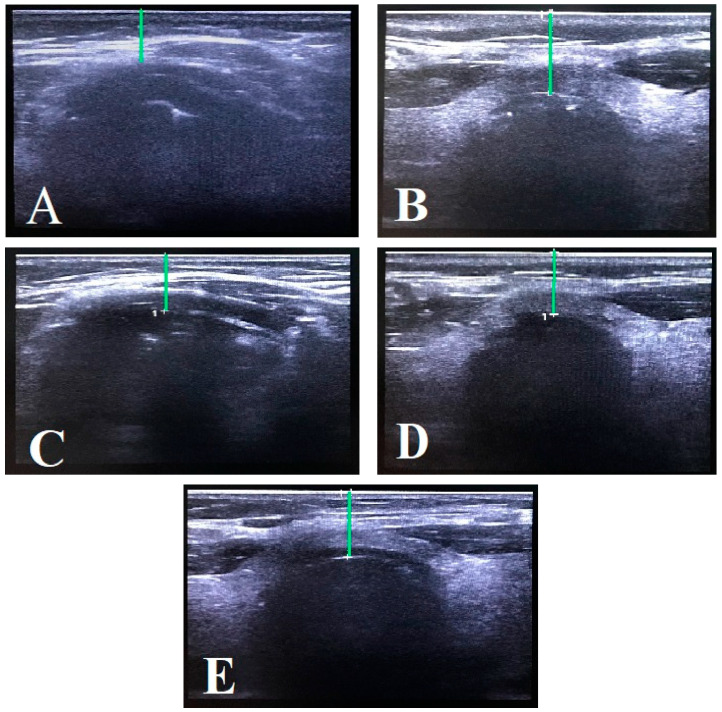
Ultrasonography distances: (A) DSHB. (B) DSEM. (C) DSAC. (D) DSTI. (E) DSTJ.
